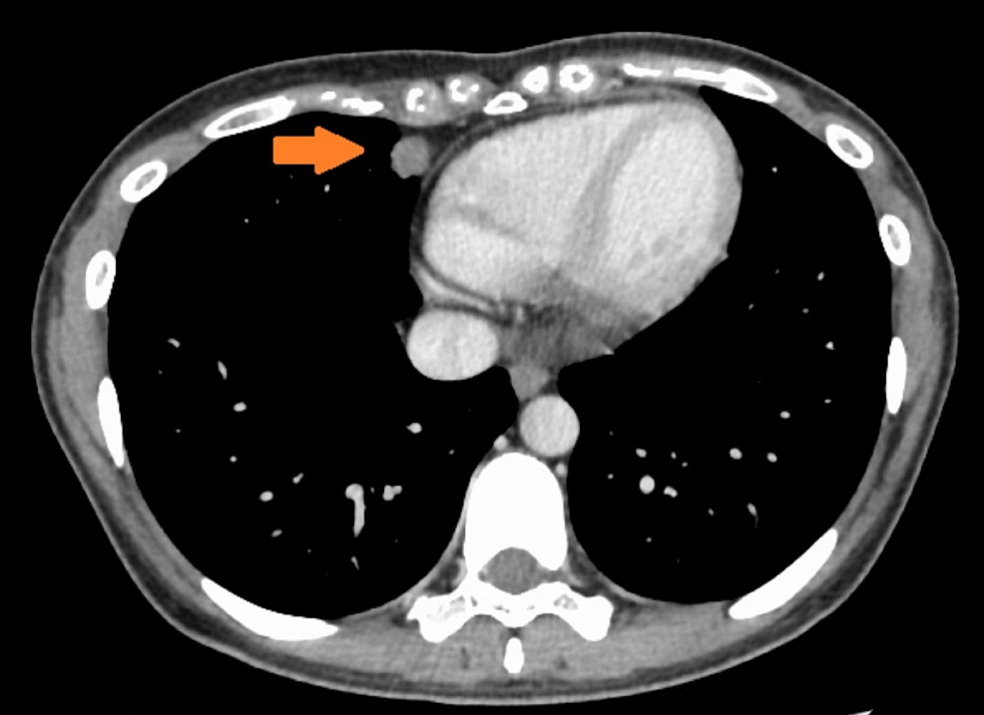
CT scan of the chest. The primary site at the initial examination.
